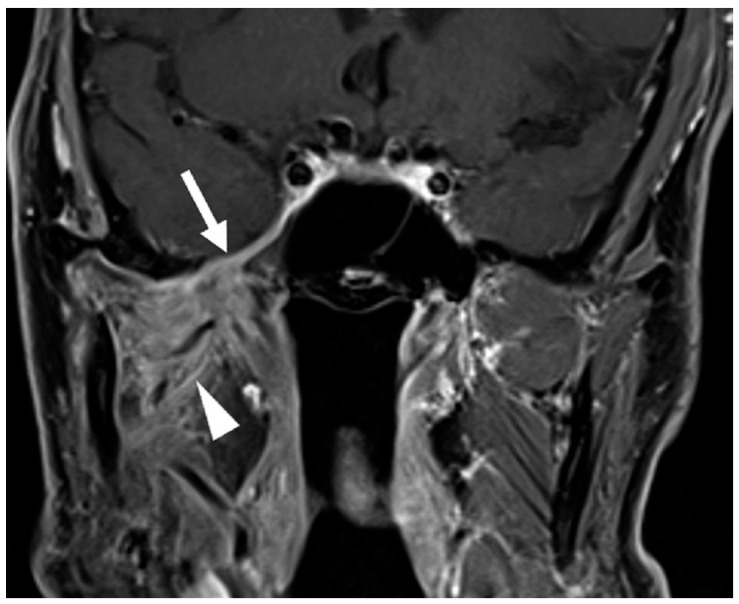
Post-treatment Examination Following Neoadjuvant Immunotherapy and Definitive Radiotherapy. Coronal T1-weighted, fat-saturated postcontrast image demonstrates decreased thickening and enhancement of the mandibular division of the right trigeminal nerve through the foramen ovale and right cavernous sinus (arrow, compared to Figure 2B). Note persistent abnormal enhancement of the right muscles of mastication (arrowhead) consistent with denervation changes; atrophy of the muscles did not resolve post-treatment.
